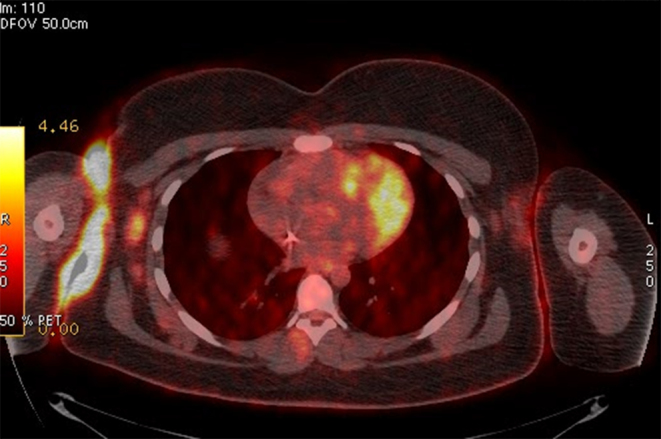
Axial fused positron emission tomography/CT image shows right axillary hypermetabolic adenopathy as well as marked skin thickening associated with hypermetabolic activity at the opposing skin folds of the right axillary region.
